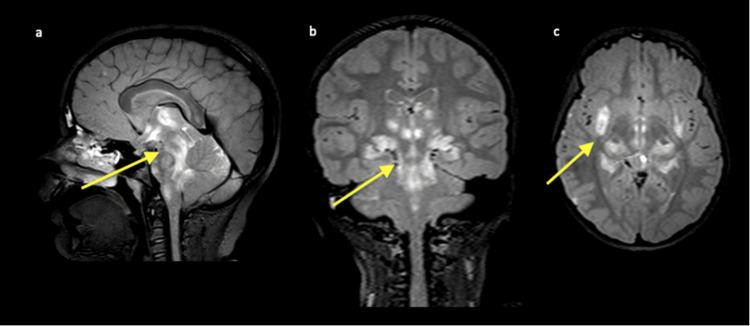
Sagittal (a), coronal (b), and axial (c) 3D FLAIR weighted images demonstrating diffuse brain swelling particularly involving the brainstem and cerebellum with narrowing of the basal cisterns and cerebral sulci as well as multifocal areas of FLAIR hyperintensity (indicated by yellow arrows) involving the lateral basal ganglia, putamen, medial thalamus and hypothalamus, midbrain, pons, medulla, hippocampus, and bilateral cerebellum. FLAIR: Fluid-attenuated inversion recovery; 3D: three-dimensional
